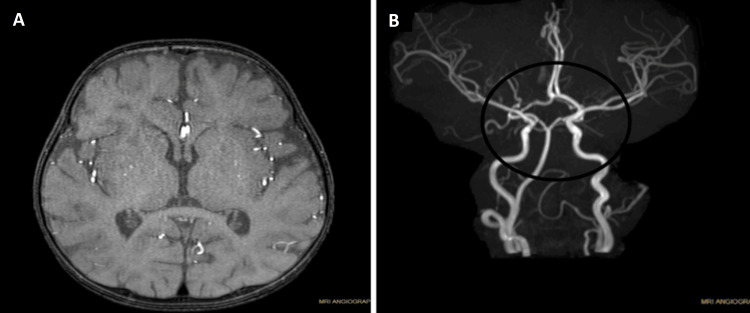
CEMRI and MRA of the patient. (A) CEMRI findings indicating multiple non-enhancing areas in various regions of the brain suggesting the presence of viral encephalitis and (B) the finding of hypoplasia on MRA brain suggesting a congenital variation in the development of the arterial system. CEMRI: contrast-enhanced magnetic resonance imaging; MRA: magnetic resonance angiography
